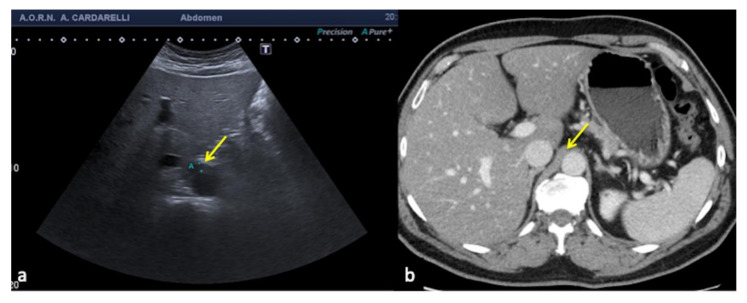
Transverse US scan of the epigastrium (a) shows a focal thickening of the aortic wall (arrow) misinterpreted as hematoma in a 45-year-old man investigated for high-dynamic deceleration trauma. On CT scan (b) it appears to be a hypertrophic right diaphragmatic pillar (arrow).
